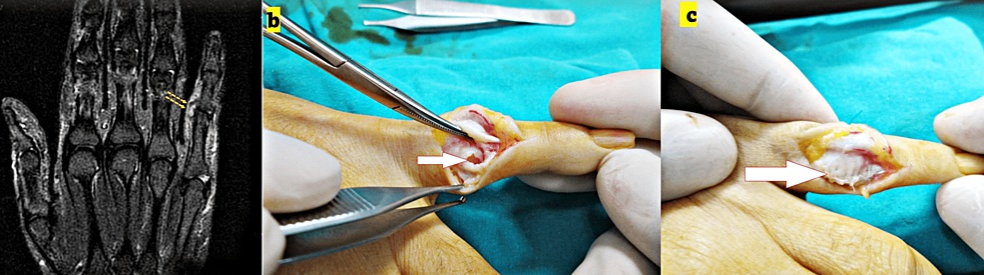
Preoperative MRI and intra-operative photograph of fingers (a) Preoperative MRI image, a coronal T2-weighted image showing the radial collateral tear of the fifth finger (yellow arrows) located in the joint proximal interphalangeal (PIP). The MRI images were reported as total rupture and verified during surgery. (b,c) Intra-operative photographs showing the repaired ligament.
